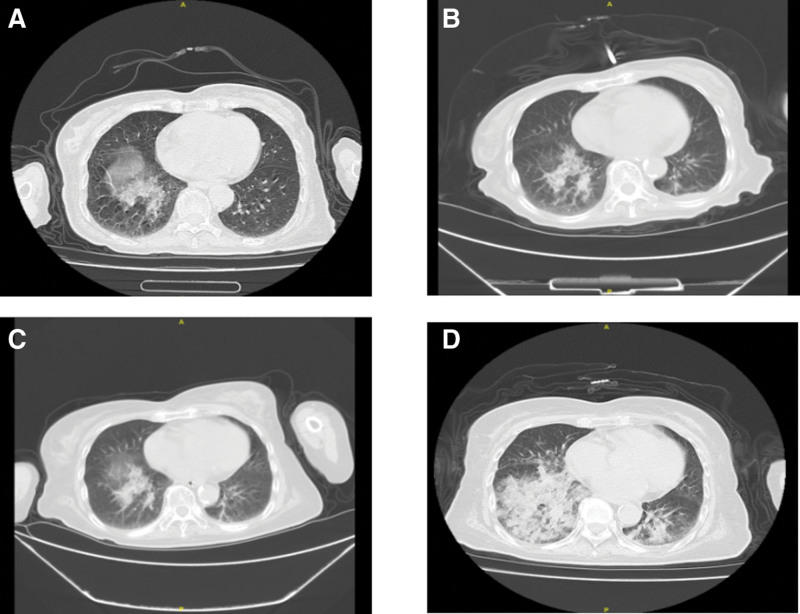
Chest computed tomography scan results of patients from June 2022 to May 2024. The results showed that multiple patchy shadows could be seen in the lower lobes of both lungs with blurred edges and uneven ends. (A) June 12, 2022. (B) December 27, 2022. (C) September 09, 2023. (D) May 04, 2024.
